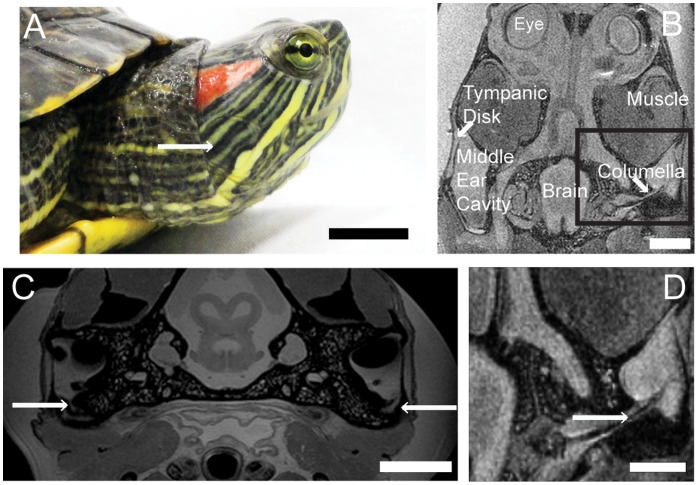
Anatomical structures of the testudine audiotory system in *Trachemys scripta elegans*. A. Lateral view of head (1 cm scale bar). B: Horizontal MR image. (500 mm scale bar) C: Transverse MRI at the level of the tectum. Arrows indicates Eustachian tubes (500 mm scale bar). “Muscle” is the splenius capitus. D: Horizontal MR image, enlarged from box in B. The columella runs through the middle ear cavity to the inner ear. Arrow indicates the columella (500 mm scale bar).
